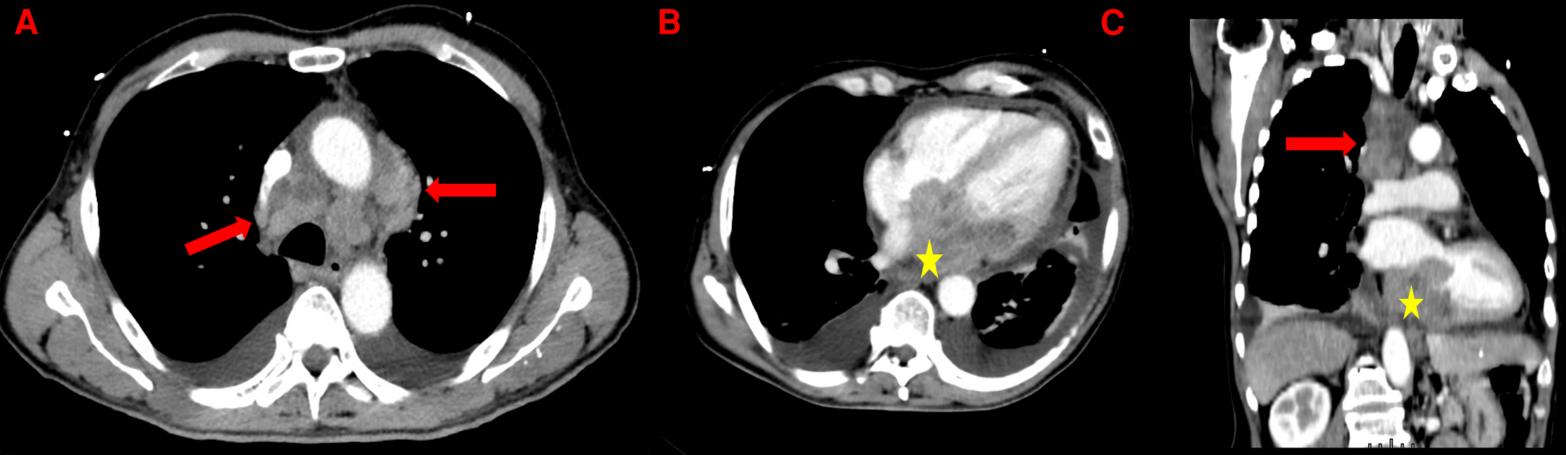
Thoracic CT-scan showing mediastinal lymphadenopathies (red arrows) and the left atrial mass (yellow stars) in axial view (A and B) and in coronal view (C). Presence of a bilateral pleural effusion and a thickened pericardium (B).
